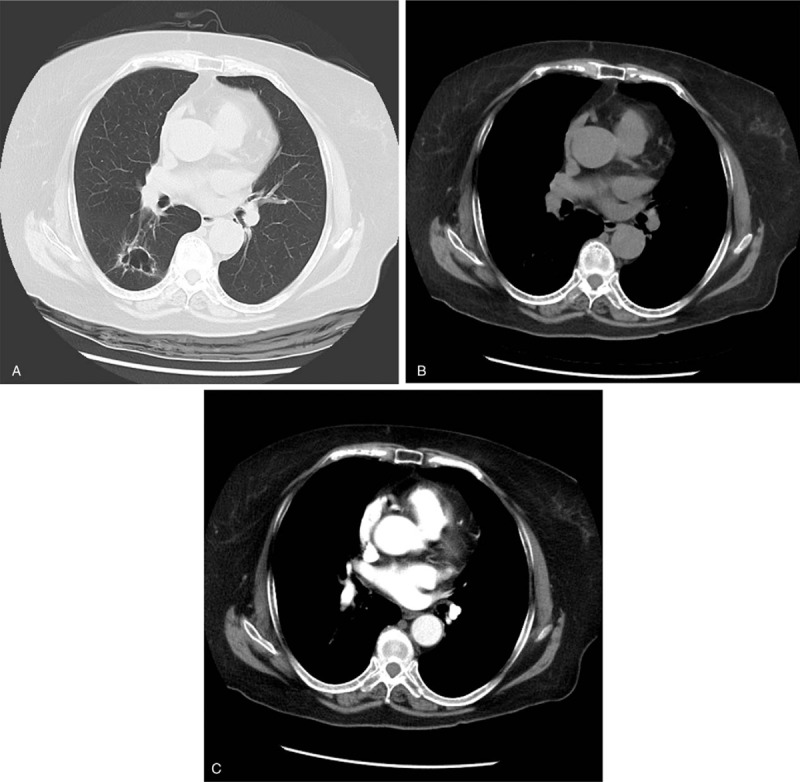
(A–C) Chest radiography showed a 35 × 22 mm cystic lesion in the right lower lung. Lung window setting (A), soft tissue window setting (B), and contrast enhanced chest CT (C). CT = computerized tomography.
